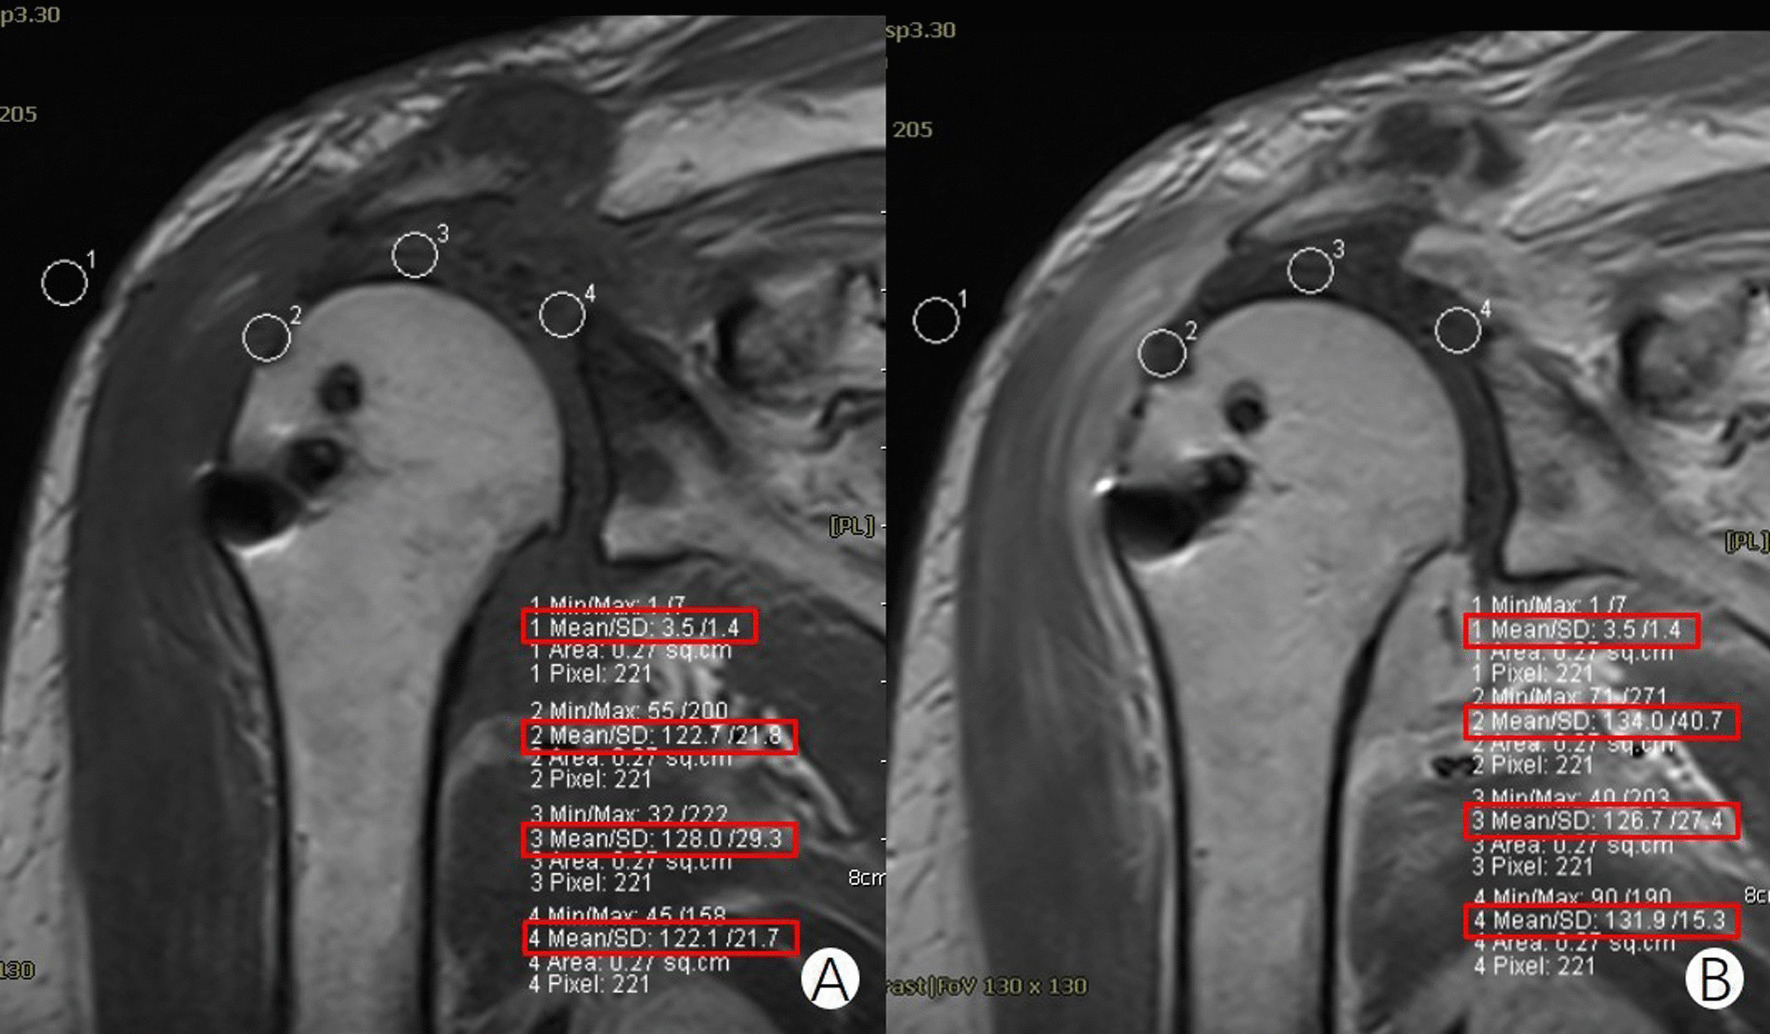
SNQ values were measured in T1WI plain magnetic resonance imaging (MRI) and enhanced MRI scans. A: T1WI-TSE-coronal plain scan; B: Enhanced T1WI-TSE-coronal position. Red rectangle 1 in both A and B shows that the signal values in the blank areas of the plain scan and enhancement phases were the same. Red rectangles 2, 3, and 4 show the measurement of signal intensity at three different positions (GTI, MG, and GI)
